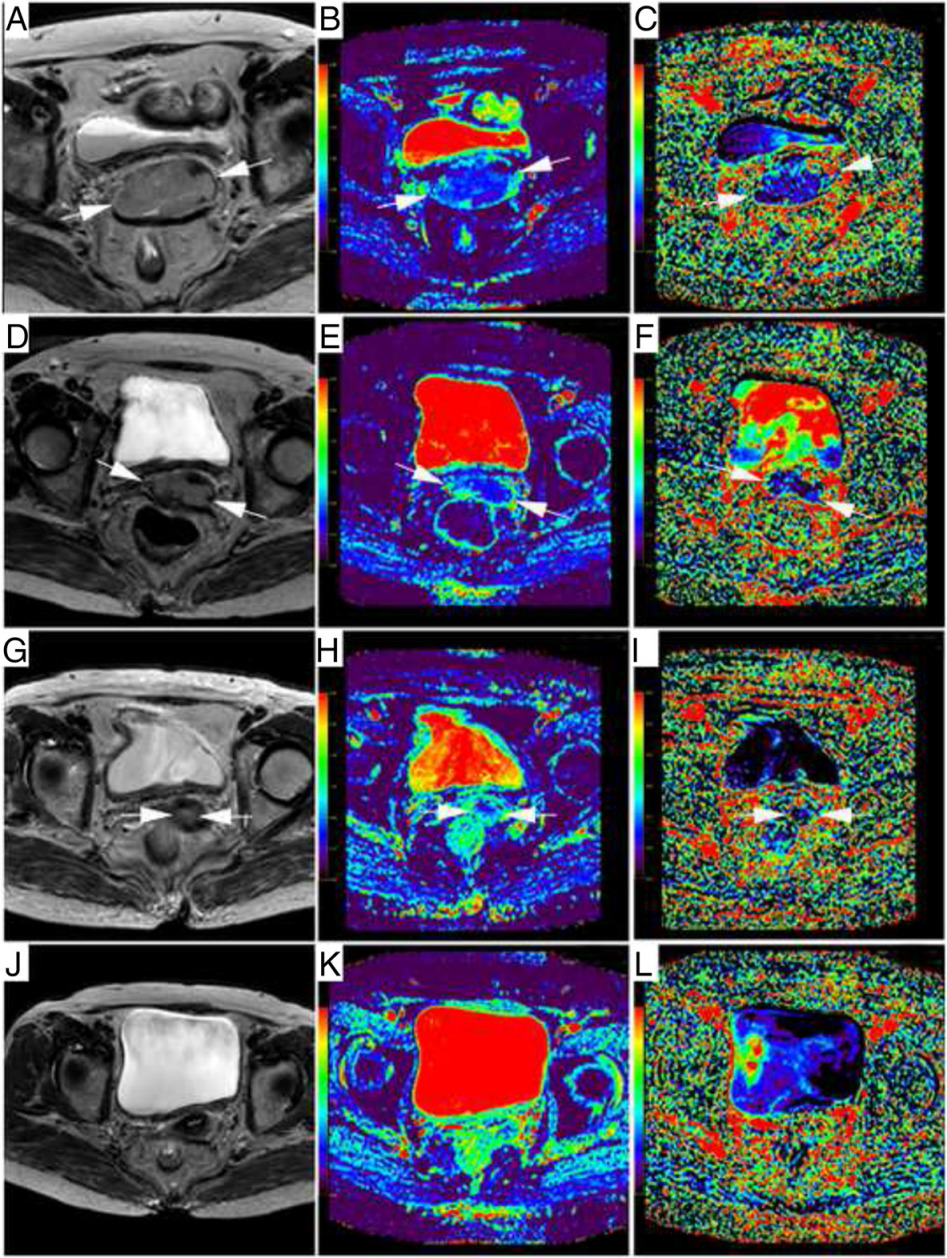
MR images of a patient receiving concurrent chemo-radiotherapy (CCRT) for advanced cervical cancer (FIGO stage IIIA). a Axial T2-weighted image before CCRT shows a hyperintense mass lesion (arrows) at the cervix, with a maximal diameter of 6.0 cm. b ADC map corresponding to (a) shows a low ADC value (0.933 × 10^−3^ mm^2^/s) of the lesion compared with the adjacent normal cervical tissues (1.54 × 10^−3^ mm^2^/s). c The *f* map corresponding to (a) shows a low *f* value (0.097) of the lesion compared with the adjacent normal cervical tissues (0.198); d Two weeks after initiation of CCRT, the diameter of cervical cancer decreased to 4.2 cm. e The ADC value of the lesion corresponding to (d) increased to 1.038 × 10^−3^ mm^2^/s compared with (b). f The *f* value of the lesion corresponding to (d) increased to 0.116 compared with (c). g One month after initiation of CCRT, the lesion continues to decrease with a diameter of 1.7 cm. h The ADC value of the lesion corresponding to (g) continues to increase to 1.563 × 10^−3^ mm^2^/s compared to (e). i The *f* value of cervical cancer corresponding to (g) continues to increase to 0.239 compared to (f). j Post CCRT, no residual lesion is observed in the axial T2-weighted image. k The ADC value of the lesion corresponding to (j) continues to increase to 1.737 × 10^−3^ mm^2^/s compared to (h). l The *f* value of cervical cancer corresponding to (g) continues to increase to 0.165 compared to (i)
